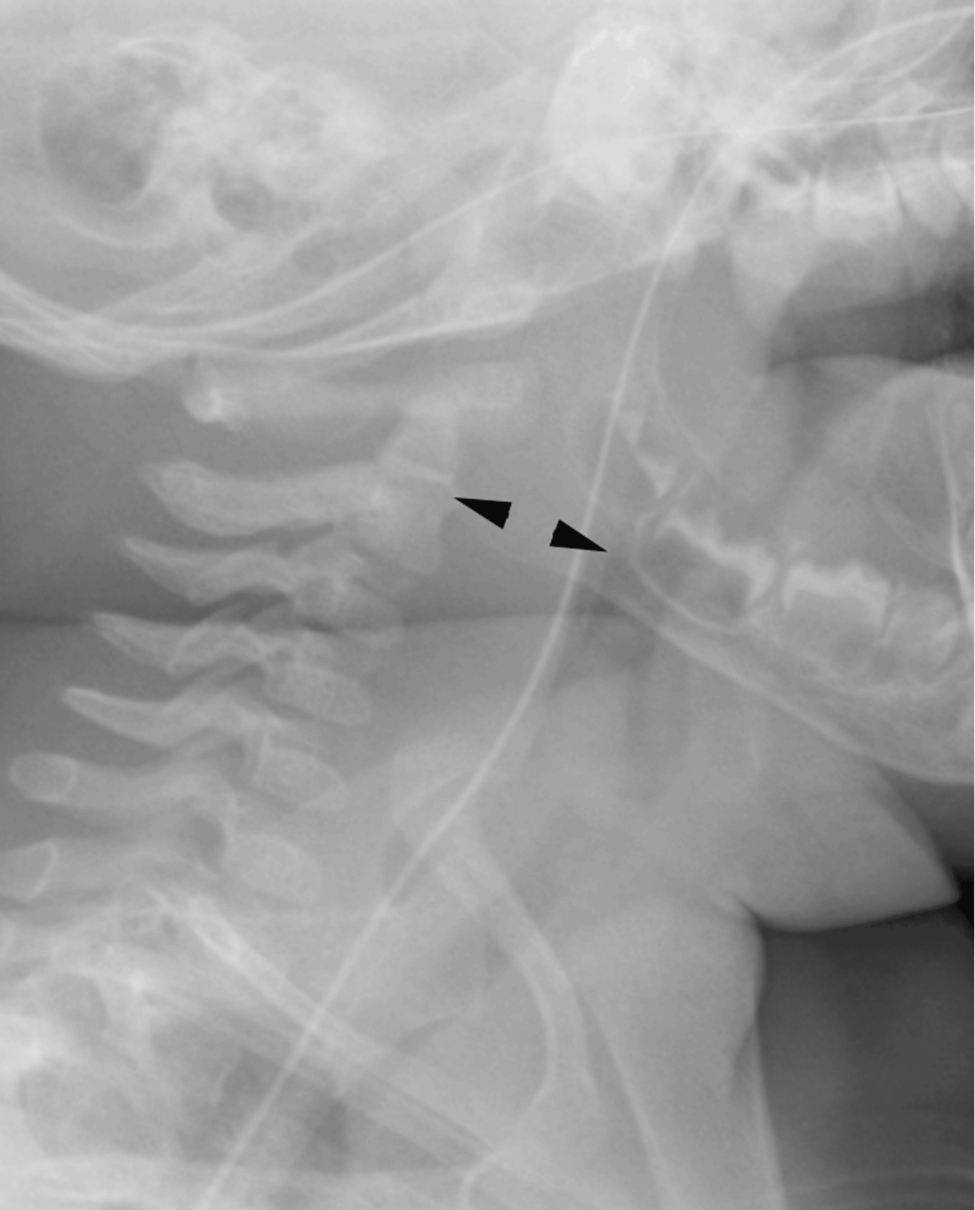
Lateral neck radiograph showing widening of the prevertebral space (arrowheads), measuring up to 13 mm at the C2 level and 14 mm at the C6 level. No gas shadows were observed. The airway was displaced anteriorly but without significant stenosis. No hyperdense foreign body or suspicious lytic cervical spinal lesions were detected.
